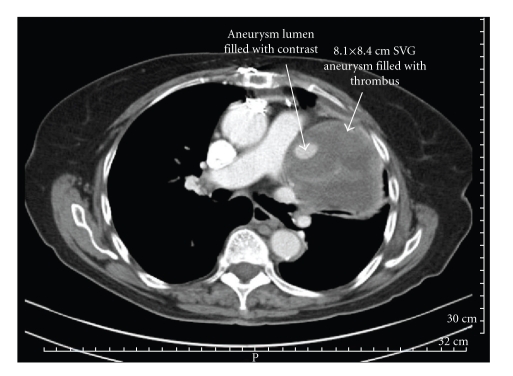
CT scan chest. An 8.1 × 8.4 cm mass is noted adjacent to left ventricle. Central filling (2 × 2 cm) of the mass with contrast is seen and is surrounded by thrombus.
